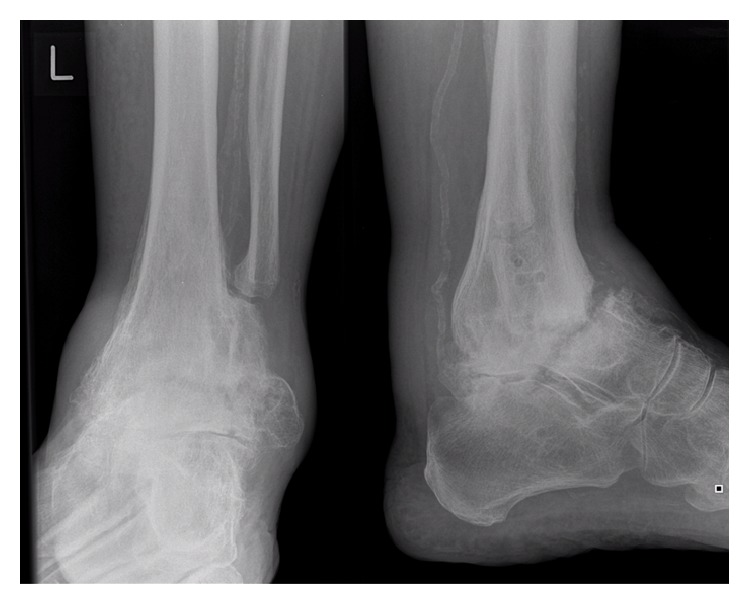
Radiograph at initial presentation to our institution, demonstrating nonunion of talar neck fracture below tibiotalar arthrodesis.
